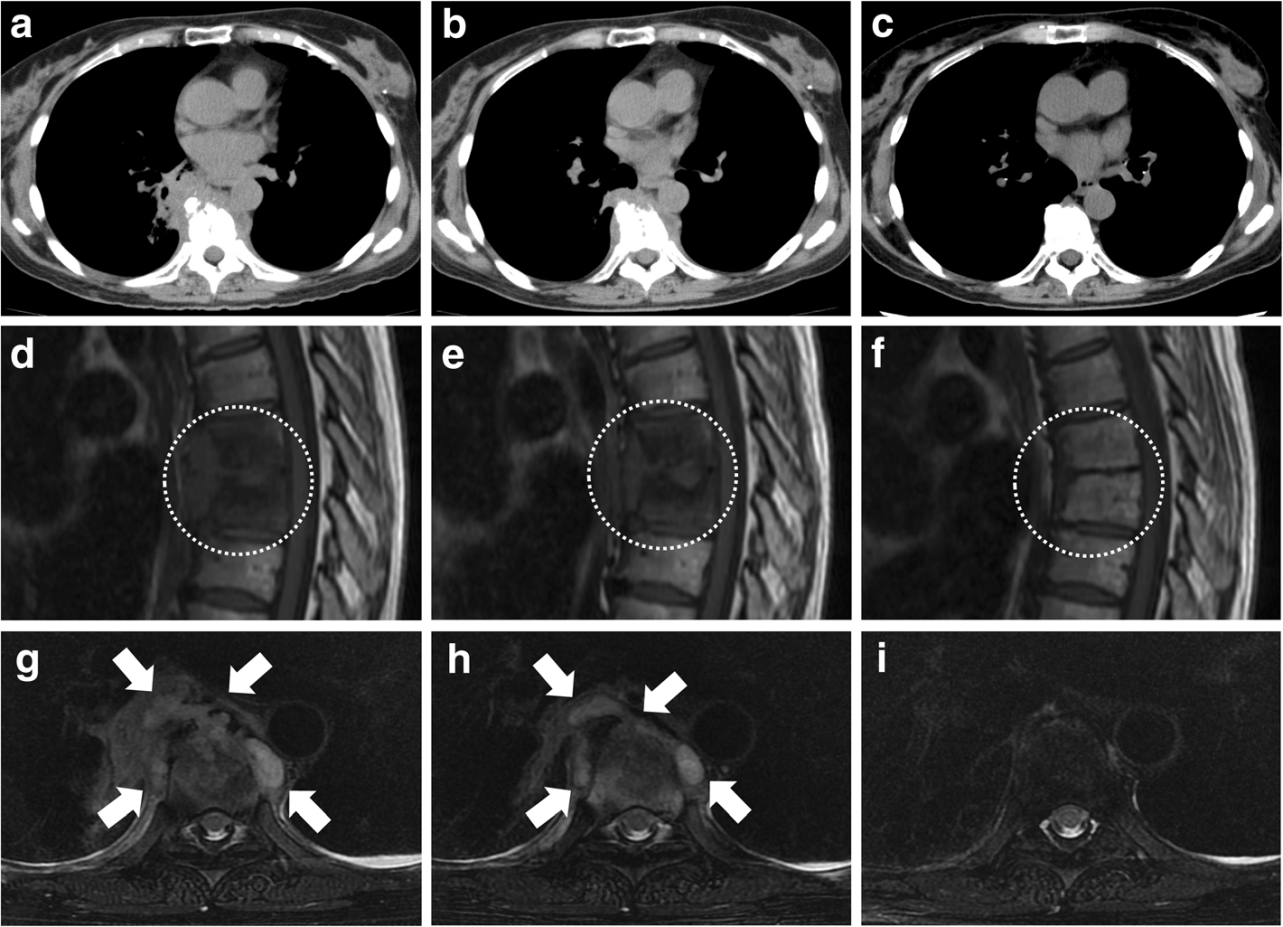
Chest CT scans (a-c) and spinal MRI (d-f T1-weighted, g-i fat-suppressed T2-weighted) obtained after initiation of antimycobacterial treatment (a, d and g at 1 month, b, e and h at 3 months, c, f and i at 2 years). Both the VO (bone destruction on CT and low signal on T1-weighted MRI (circled)) and the abscess (paravertebral lesion on CT and high signal on fat-suppressed T2-weighted MRI (arrow)) improved gradually over 2 years
